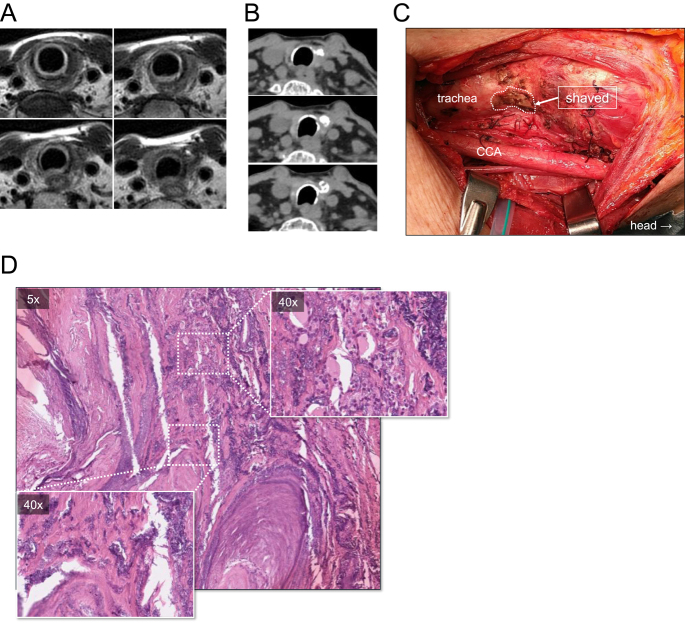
Case 12 ATC patient with airway obstructive symptoms successfully treated with bridging LEN treatment and subsequent DT, leading to conversion surgery. (A and B) Neck MRI and CT scan images after 56 days of LEN treatment and subsequent DT treatment (6 (MRI) and 8 (CT) months) indicate dramatic response to treatment, and the area of tracheal invasion was no longer detectable. (C) Intraoperative image shows shaved trachea for tumor involvement. Intraoperative frozen section diagnosis of the invasive front in the tracheal wall revealed non-viable atypical cells. Dotted line indicates shaved area. (D) Final pathological diagnosis was CR with nodular changes consisting of fibrosis, hyalinization, coarse calcification deposits, and foamy cells. Regenerative follicles in the surrounding area and some roundly arranged epithelial cells without atypia were observed.
